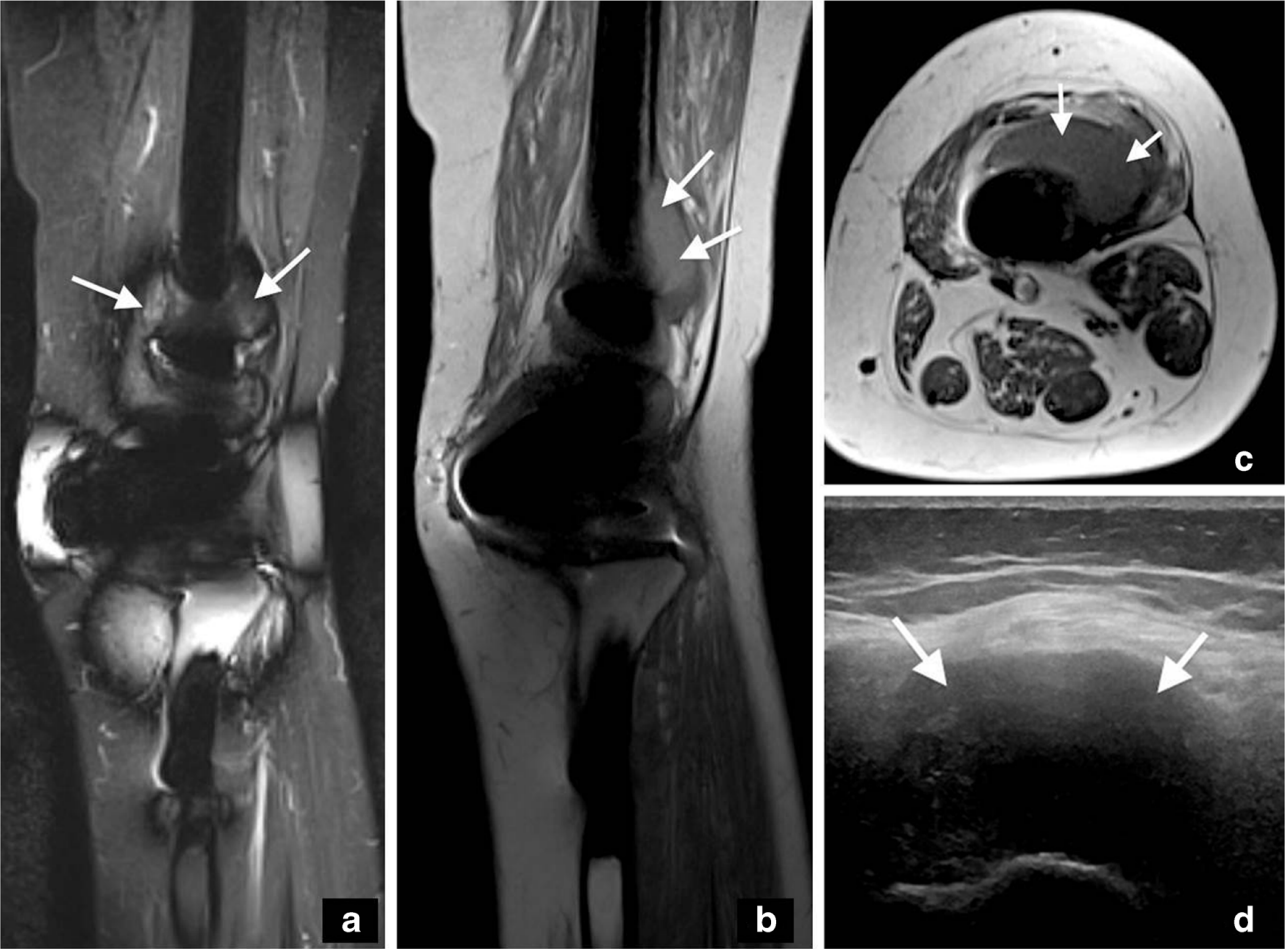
a Coronal STIR, b coronal T1, c axial T1-weighted MRI and d axial ultrasound image 6 years post-index surgery show a complex, predominantly thin-walled cystic soft-tissue mass intimately related to the antero-lateral aspect of the femoral component of the prosthesis (*arrows*). The lesion is well-defined, returns largely low STIR and is isointense to high T1-weighted signal relative to skeletal muscle, consistent with a pseudotumour. The diagnosis is supported by the homogeneous, hypoechoic appearance on ultrasound, with no Doppler activity
